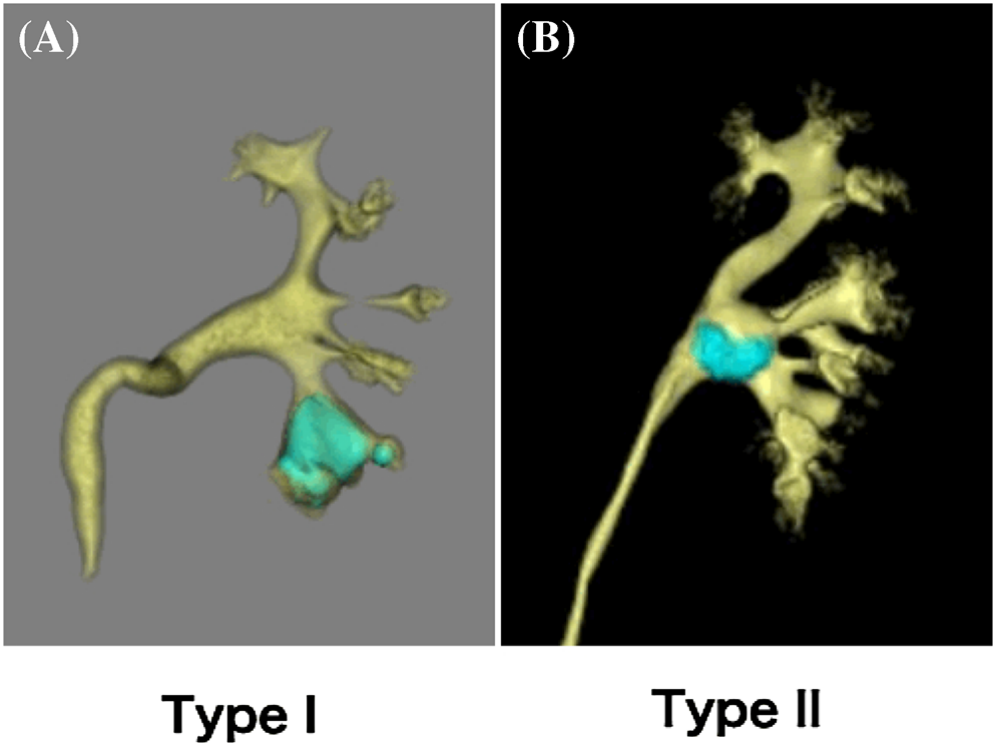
Takazawa classification of the pelvicalyceal anatomical system of representative images of CT urography. (A) Type I, (B) Type II
